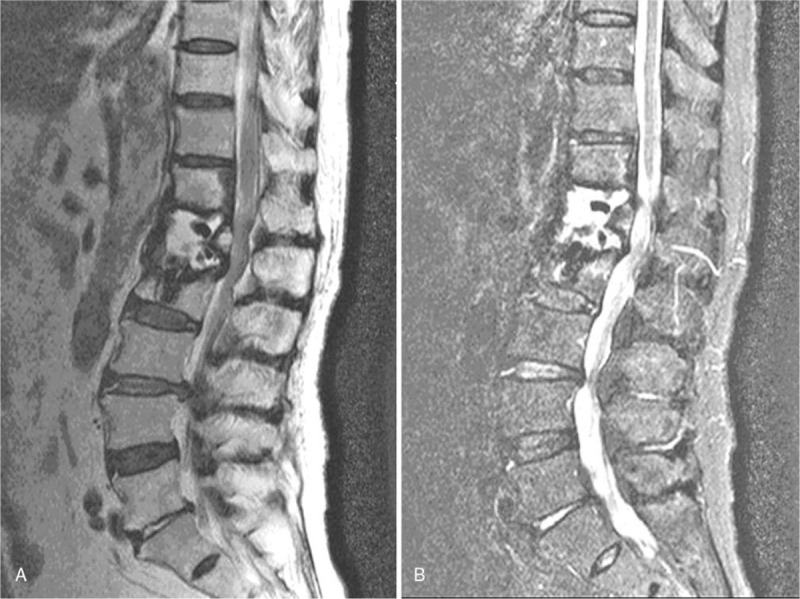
Sagittal T1-weighted and STIR MR images (Magnetom expert 1.5 Tesla) showing low and high signal intensity on L1, respectively.
